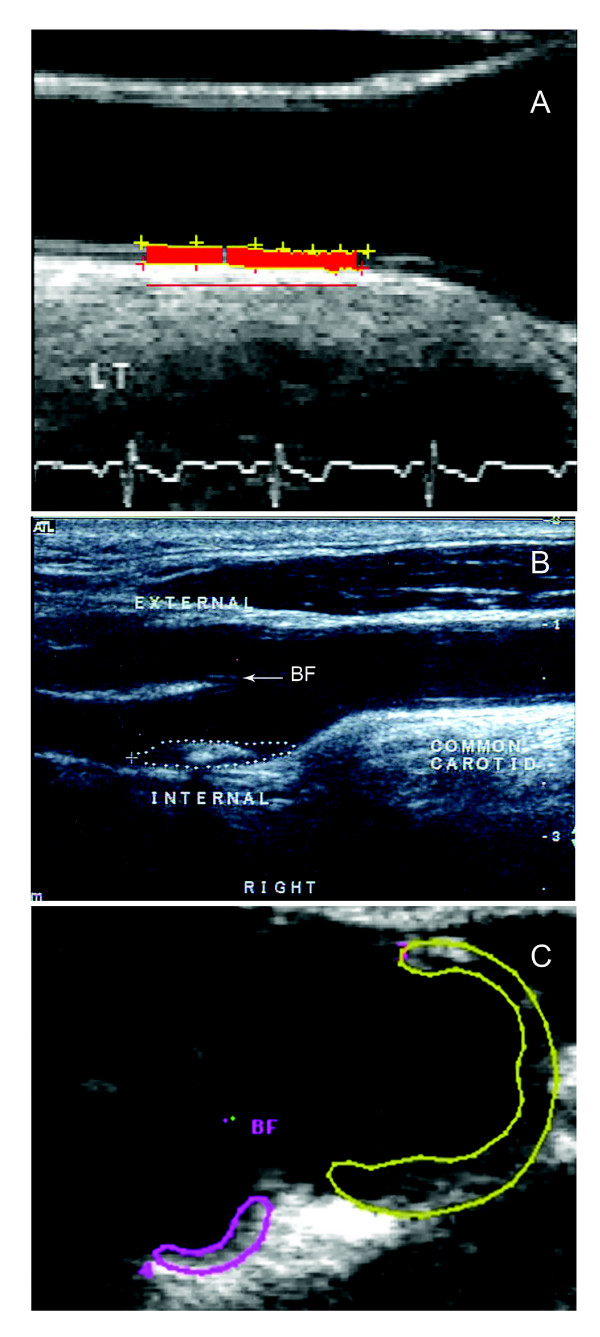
Carotid atherosclerosis imaging phenotypes. A) IMT measurement from a B-mode ultrasound image in the longitudinal view with the carotid bifurcation identified as BF. B) TPA measurement from a longitudinal B-mode US image with the outlined plaque area shown in white. C) cross-sectional or axial view of the internal and external carotid artery with manual TPV measurements in both branches of the artery and the bifurcation marked BF.
